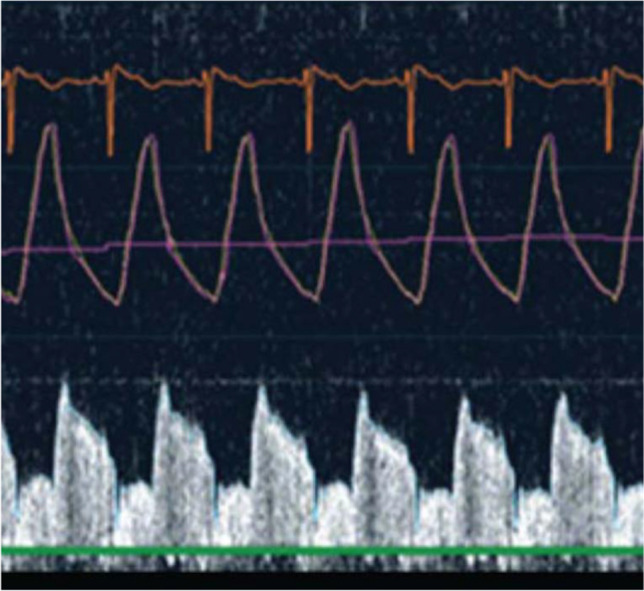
Doppler wire tracing. A Doppler-tipped guidewire allows direct measurement of coronary flow velocity. Doppler peak flow velocities are averaged over three consecutive heartbeats at rest and during hyperemia to calculate resting and hyperemic average peak velocities, respectively
